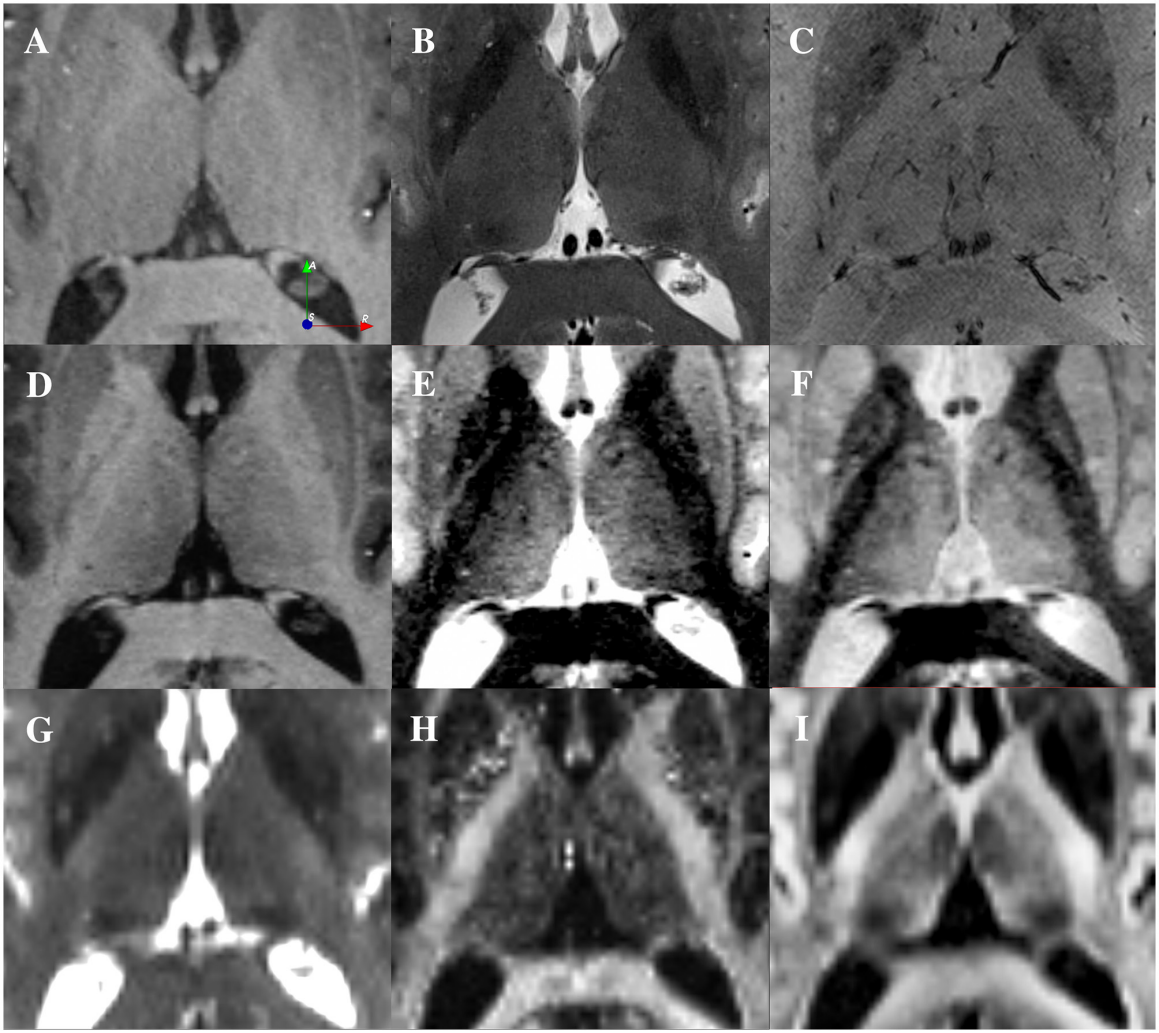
Visualization of the thalamus using different contrasts at 7T from a single participant. (A) T1-weighted image (0.6 mm isotropic). (B) T2-weighted image (0.4x0.4x1 mm). (C) SWI image (0.4x0.4x0.8 mm). (D) Multi-echo MP2RAGE (0.7 mm isotropic). (E) Synthetic WMn-MPRAGE reconstructed from the multi-echo MP2RAGE (0.7 mm isotropic). (F) FGATIR image (0.8 mm isotropic). (G) Average across 4 B0 images from a dMRI dataset (1.25 mm isotropic). (H) Fractional Anisotropy computed the dMRI data (1.25 mm isotropic). (I) DiMANI image reconstructed from the dMRI dataset (1.25 mm isotropic).
